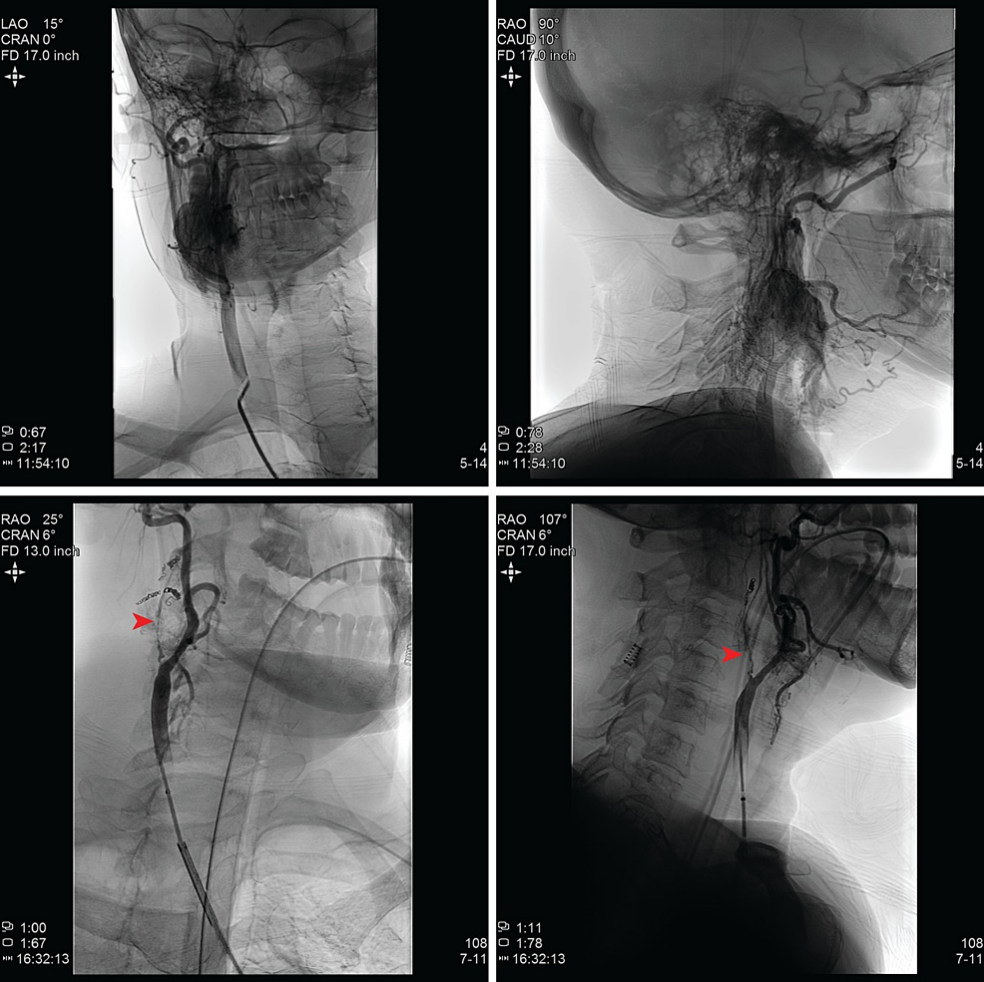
CT angiography during embolization. Rich vascular supply of the tumor (top) and the significant reduction in its blood supply after (bottom) embolization of the feeding arteries.
